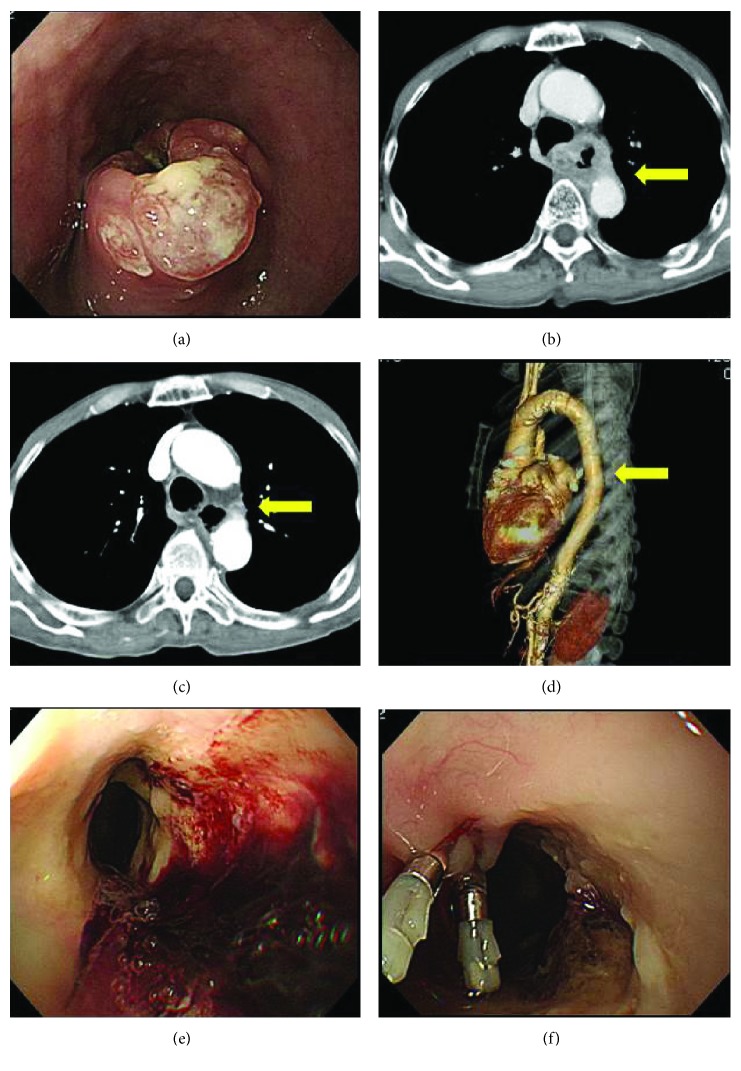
Imaging at the initial treatment for the aortoesophageal fistula using the TEVAR method. (a) EGD revealed a type 2 lesion spanning 3/4 of the circumference of the middle thoracic esophagus. (b) Computed tomography scan (CT) revealed esophageal wall thickening with tumor, and there was loss of the normal fat plane between the esophagus and the adjacent aorta. Approximately 90° of the circumference of the aorta was in contact with the tumor, which suggested aortic invasion (yellow thin arrow). (c) CT scan revealed aortic erosion of the intravenous contrast material within the descending thoracic aorta and extraluminal foci of air between the adjacent esophagus and the aorta (yellow thin arrow). (d) CT angiography did not indicate active bleeding. (e) Emergency esophagogastroduodenoscopy showed a pale fragile esophageal lesion on the posterior wall, an area previously treated with chemoradiation therapy, with massive blood coagulation. (f) Marking clips were placed on the side opposite the lesion.
